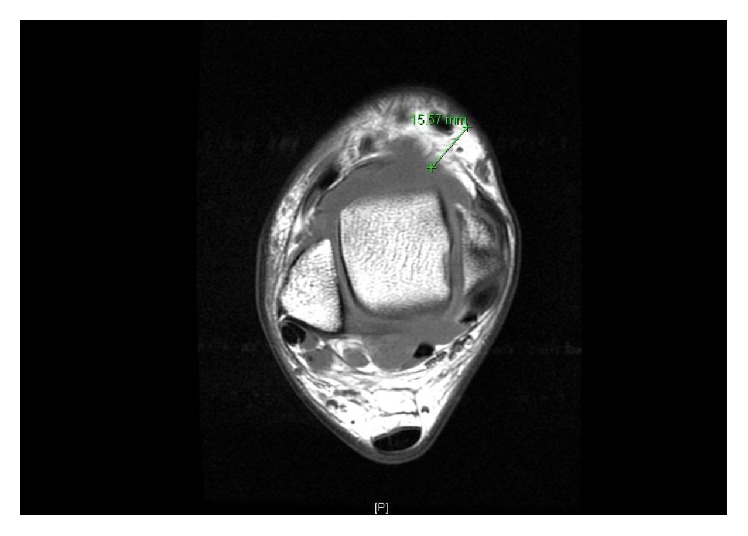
MRI showing a large (R) ankle effusion with tibialis posterior tenosynovitis.
